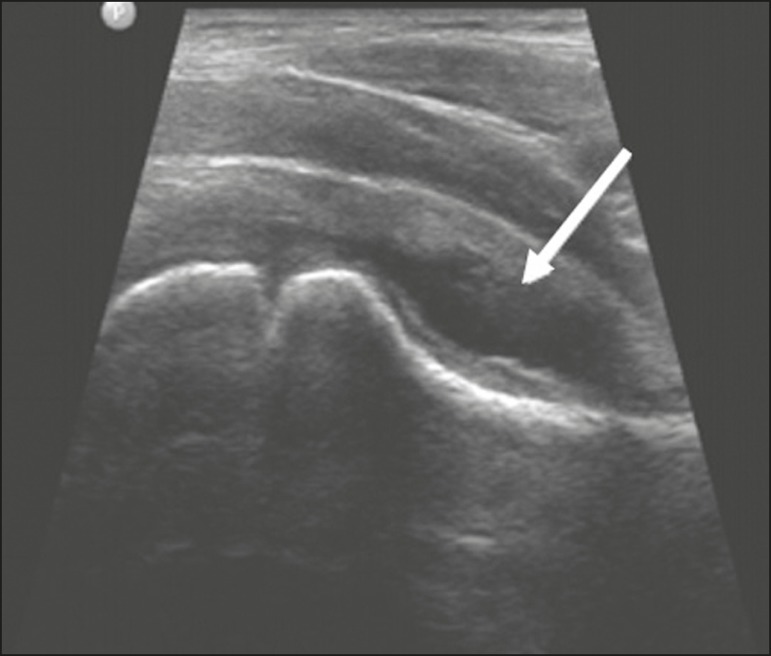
Ultrasound of the hip identifying joint effusion.
